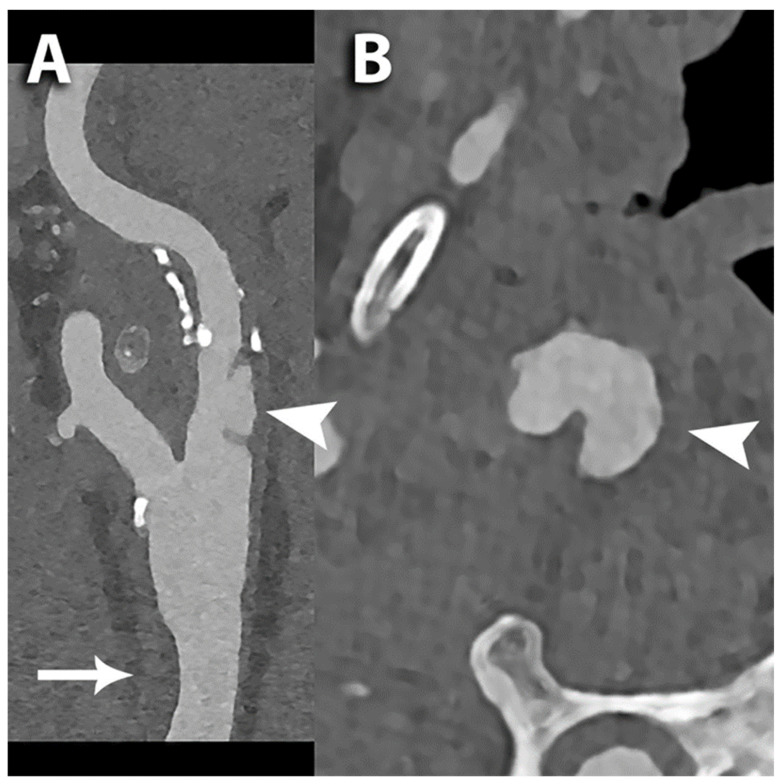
PCCT angiography of the carotid arteries; ulceration of the internal carotid artery. The figure shows a PCCT angiography of the carotid arteries and in particular a longitudinal multiplanar reconstruction (A) and an axial cross-sectional image orthogonal to the longitudinal axis of the proximal internal carotid artery (B); arrowheads in (A,B) are indicating a focal deep ulceration of the wall of the ICA; above the ulceration there is mixed plaque along the ICA; the arrow in A shows a moderate focal non-calcified eccentric thickening of the ventral portion of the distal III of the corresponding common carotid artery. The scan was performed on a commercial whole-body dual-source photon-counting CT scanner (NAEOTOM Alpha, Siemens Healthineers, Erlangen, Germany) with 0.2 mm slice thickness, 0.1 mm reconstruction increment, FOV 140–160 mm, resolution matrix of 1024 × 1024 pixels on the source axial reconstructions with a kernel filtering of Bv60-72-80 (vascular kernel sharp/ultra-sharp) and with maximum intensity of quantum iterative reconstruction (QIR 4). The displayed image resolution is 100 microns. Abbreviations: CT = computed tomography; PCCT = photon-counting CT; ICA = internal carotid artery; FOV = field of view.
